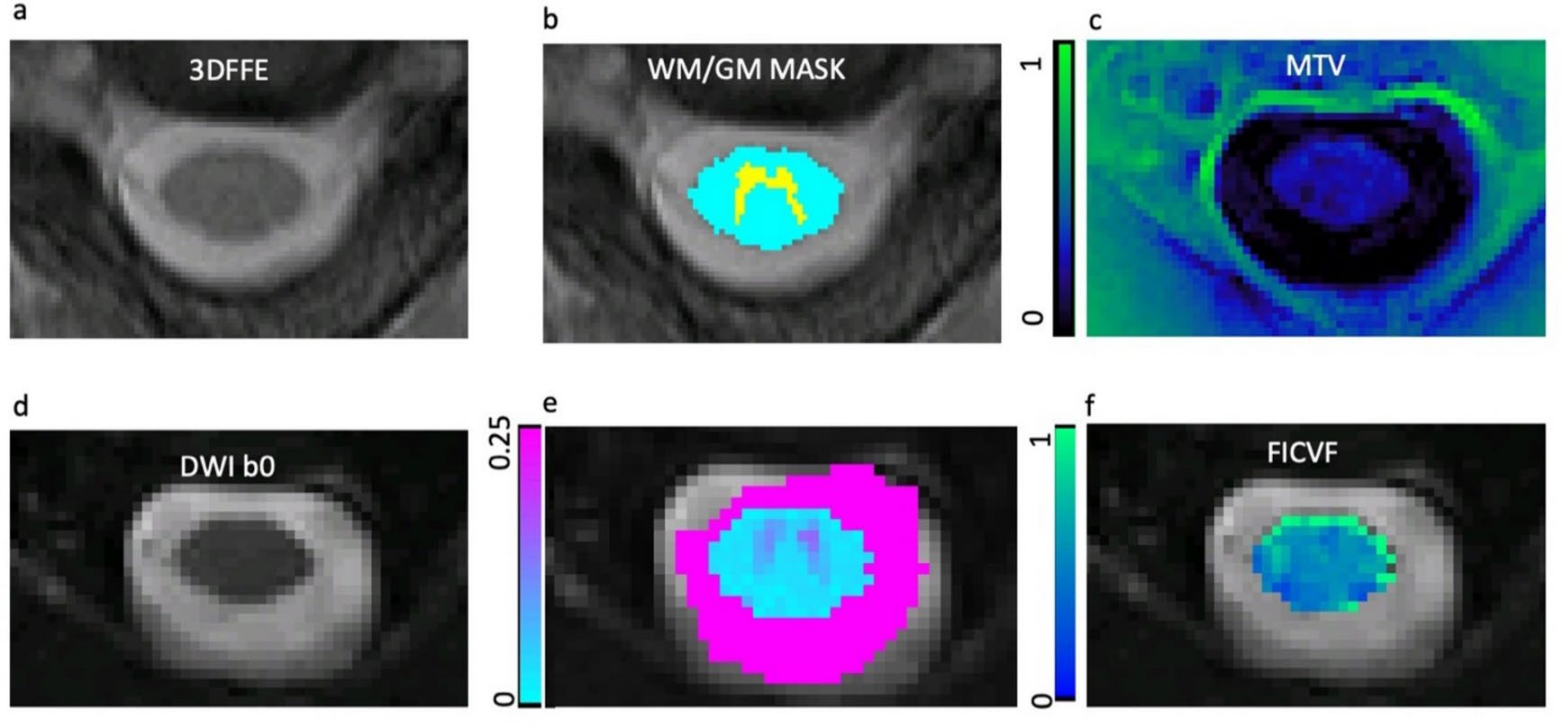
CSA segmentation, MTV and DWI maps. Example of (a) axial gradient echo image of the upper cervical cord, (b) automatic spinal cord segmentation for establishing the cross-sectional area of the upper cervical cord (WM + GM mask), (c) macromolecular tissue volume (MTV) map (d) b0 image, (e) orientation dispersion index (ODI) map, (f) intracellular volume fraction (FICVF) map.
